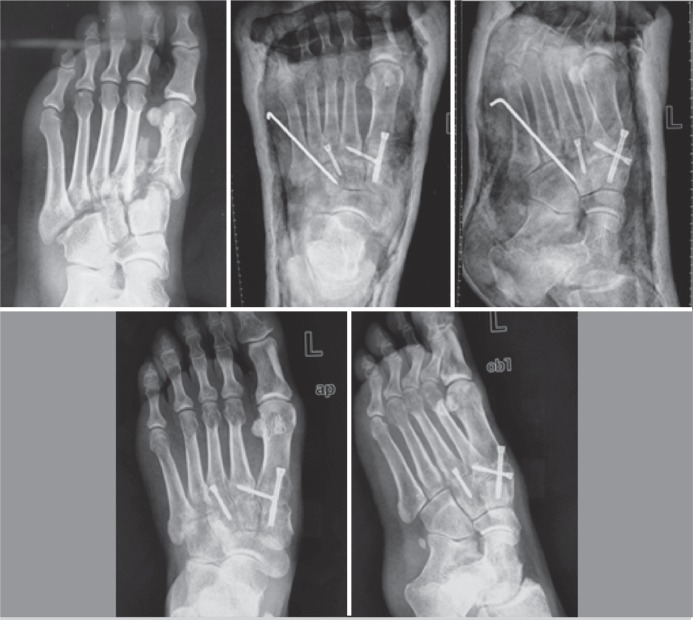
Case 2: Male 40 years old, Myerson IIB type, X-radiograph before operation; X-radiograph shows the fracture-dislocation healed two weeks and six months post-operation.
